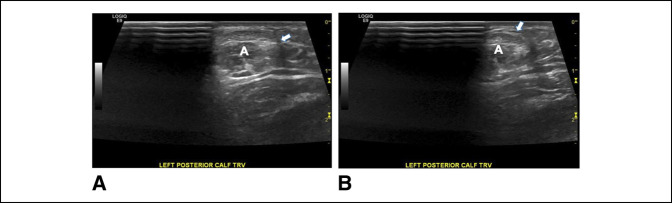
A, Transverse sonogram depicting the plantaris tendon (arrow) before subluxation medial to the Achilles tendon. B, Transverse sonogram demonstrating subluxation of the plantaris tendon (arrow) regarding the Achilles tendon (A).
